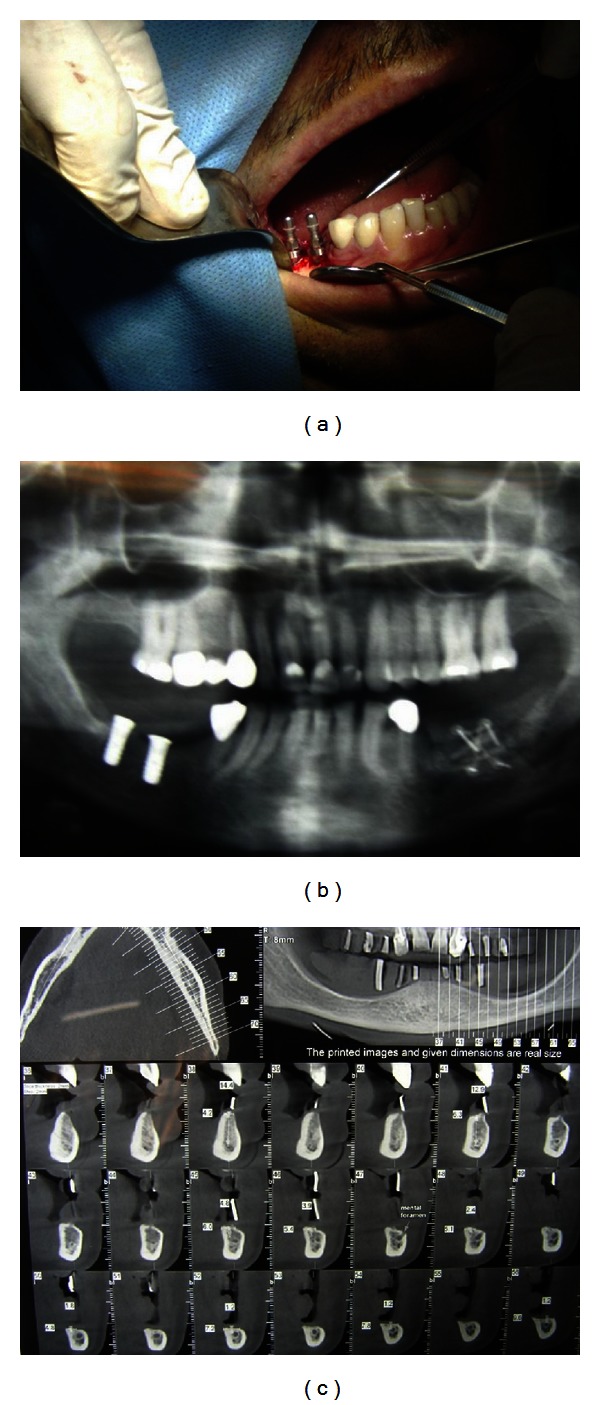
(a) Patient number one, surgery day photo. (b) Patient number one, panoramic X-ray. (c) Patient number one, CT scan.
